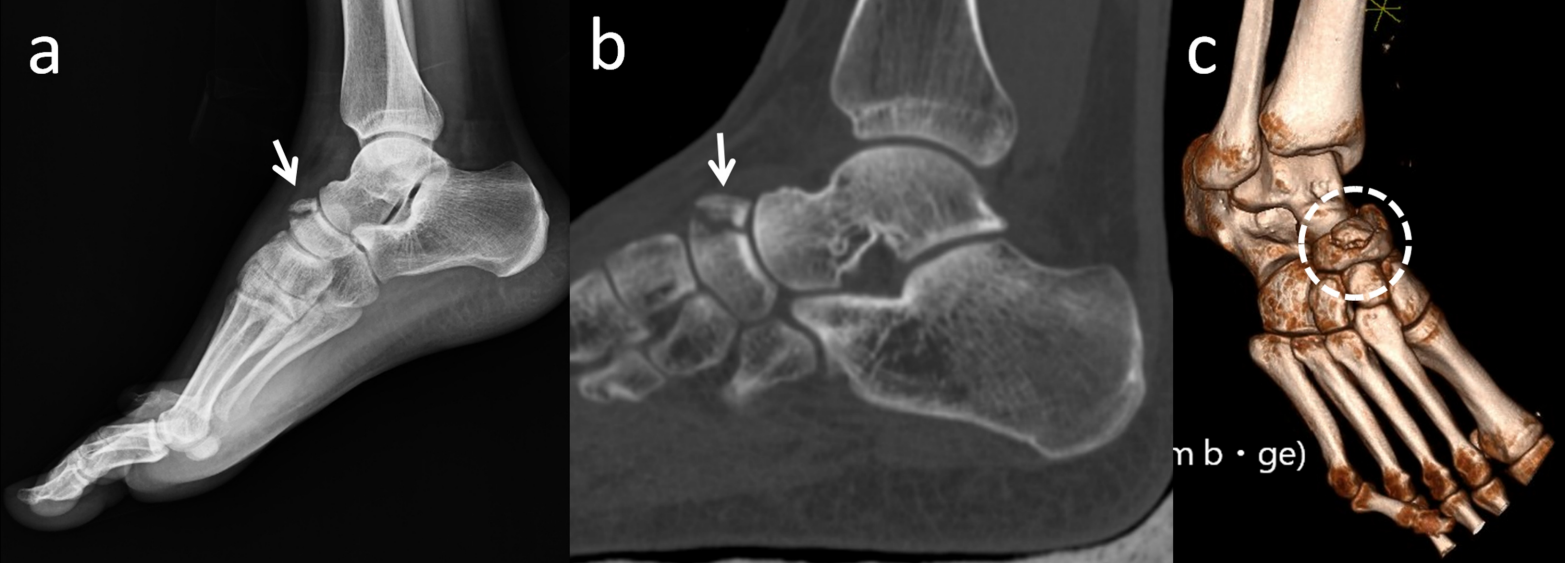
A 51-year-old female patient with an avulsion fracture of the navicular bone (a) Foot radiograph; (b) Sagittal 2D appearance (c) 3D computed tomography (CT) of the fracture - note the similarity between an os supranaviculare and an avulsion fracture.
